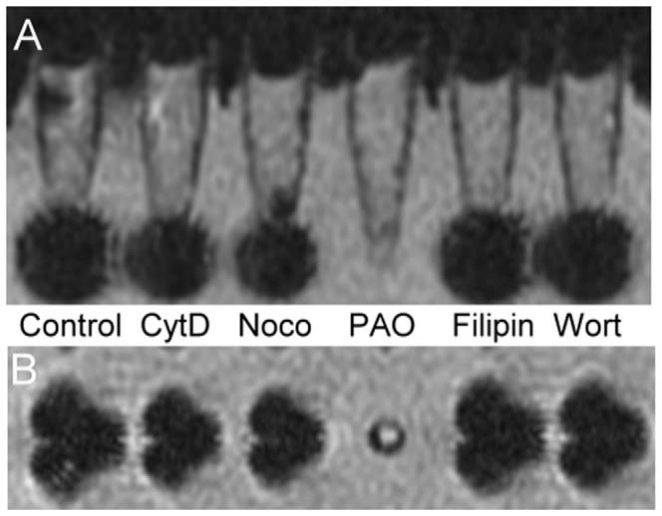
MRI of macrophages treated with different endocytotic inhibitors prior to SPIO particle uptake. (A) Coronal view and (B) axial view under T2 weighted scanning protocol. Cells were centrifuged to the bottom of the test tube and imaged as dark signals. The coronal view and axial view of these cells are consistent. There was a drop in signal intensity in the cells treated with Ferucarbotran and inhibitors, including cytochalasin D (CytD), nocodazole (Noco), filipin, and wortmannin (Wort). In contrast, the signal intensity of the cells treated with phenylarsine oxide (PAO) revealed no loss in signal intensity.
